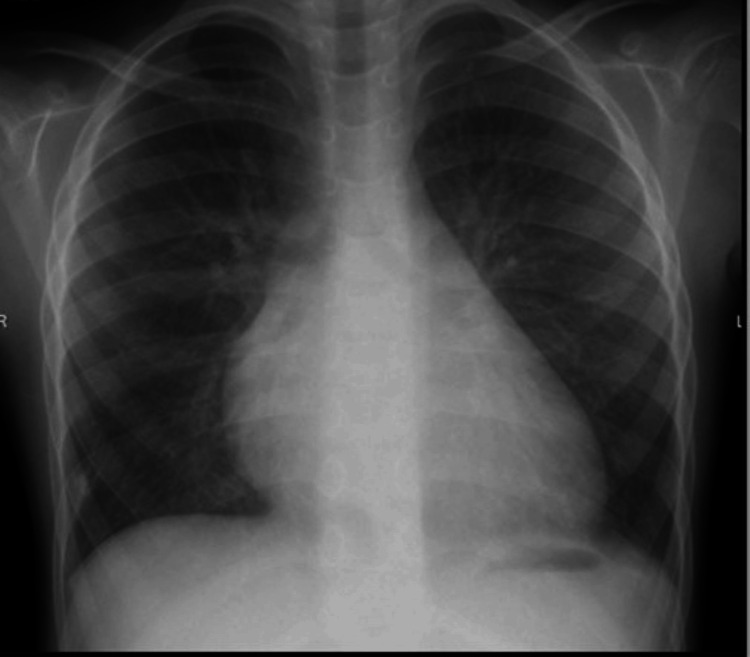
Postoperative anteroposterior chest X-ray showing cardiomegaly and residual mild pulmonary vascular congestive changes.
